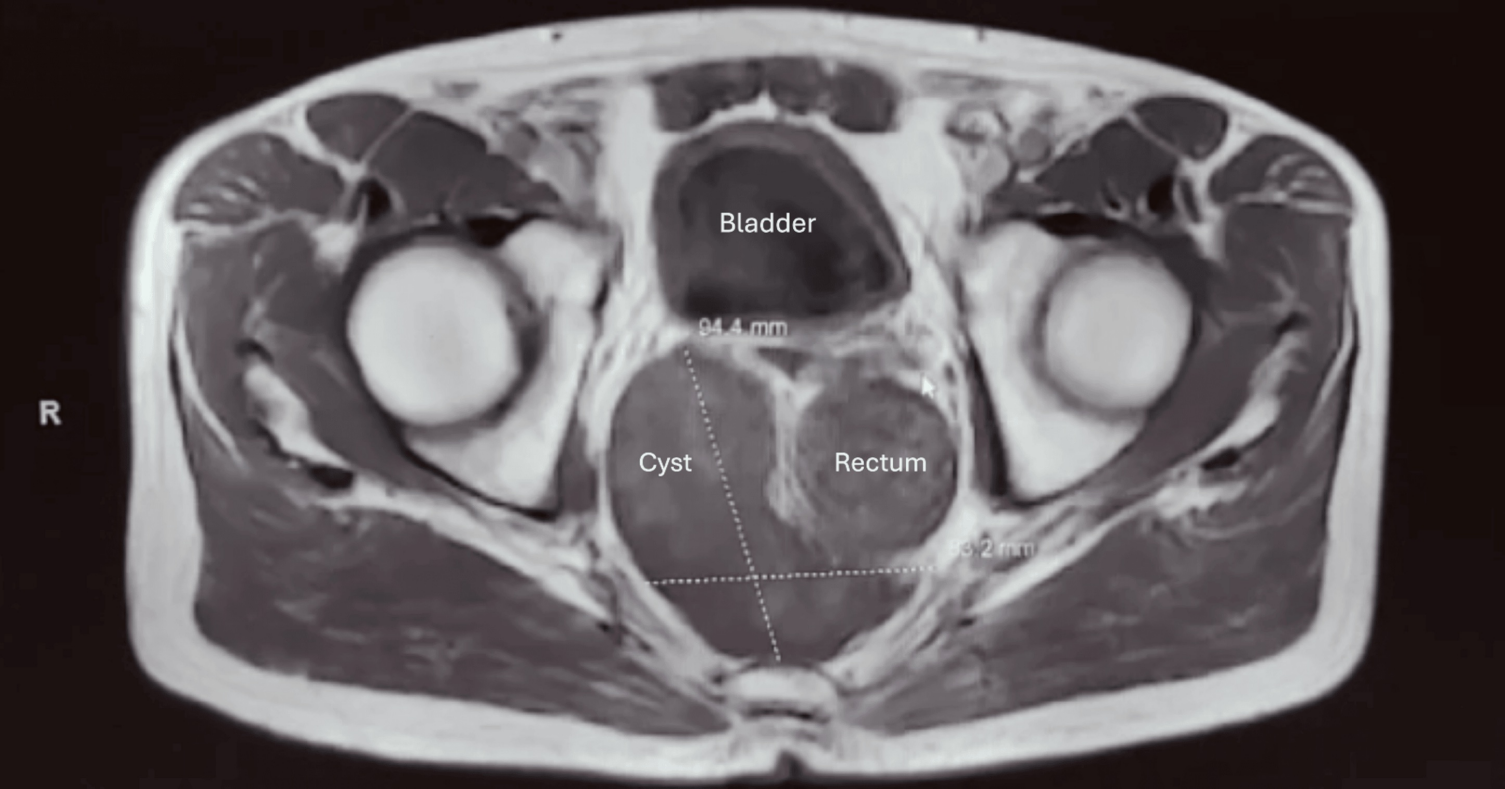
MRI image showing measurements of the patient’s presacral mass MRI: magnetic resonance imaging
